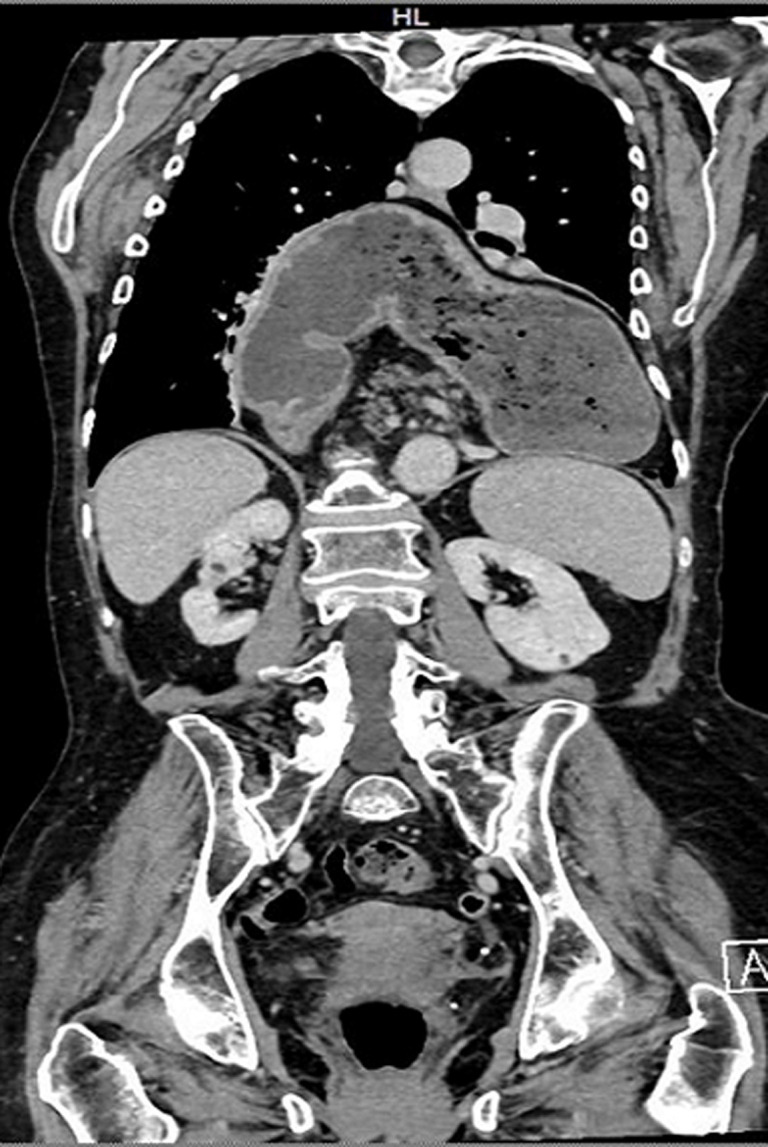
computed tomography revealed a giant hiatal hernia with stomach, ileum and colon in thoracic cavity
